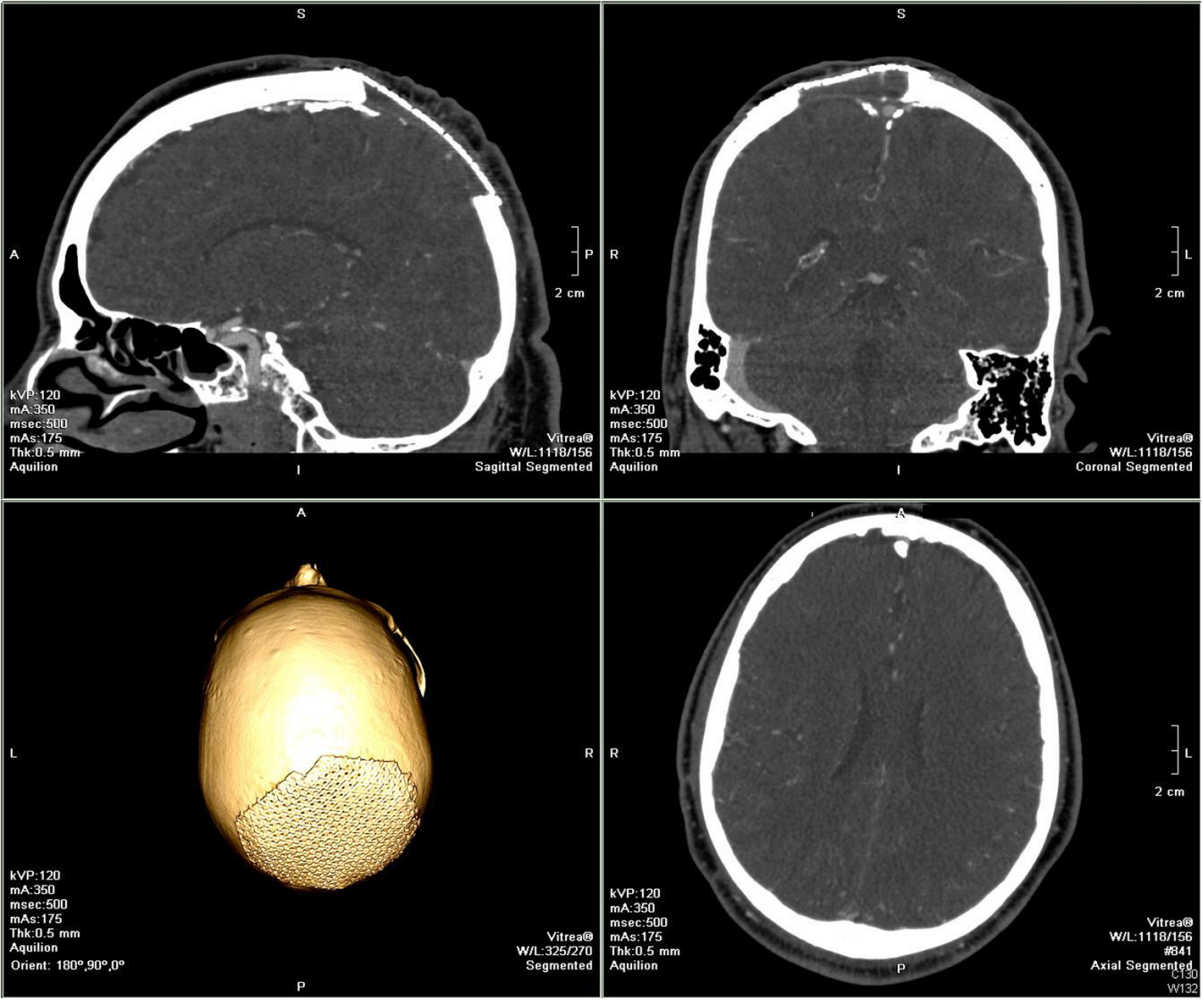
Six-month follow-up computed tomography scan with three-dimensional reconstruction. The cranial defect is shown covered by a titanium mesh. No signs of a local relapse of plasmotcytoma are evident.
